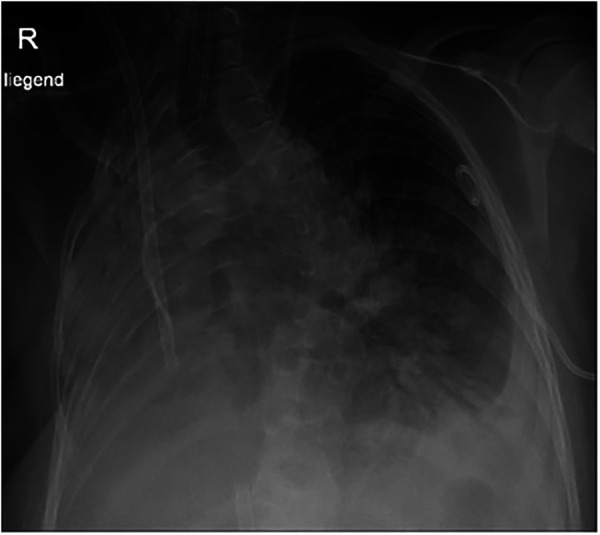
Chest x-ray at the age of 13 years 10 months: tracheal tube, ECMO tubes, left thoracic pigtail drain. Volume loss and opacification of the right lung. Basal pleural effusion on the left.
